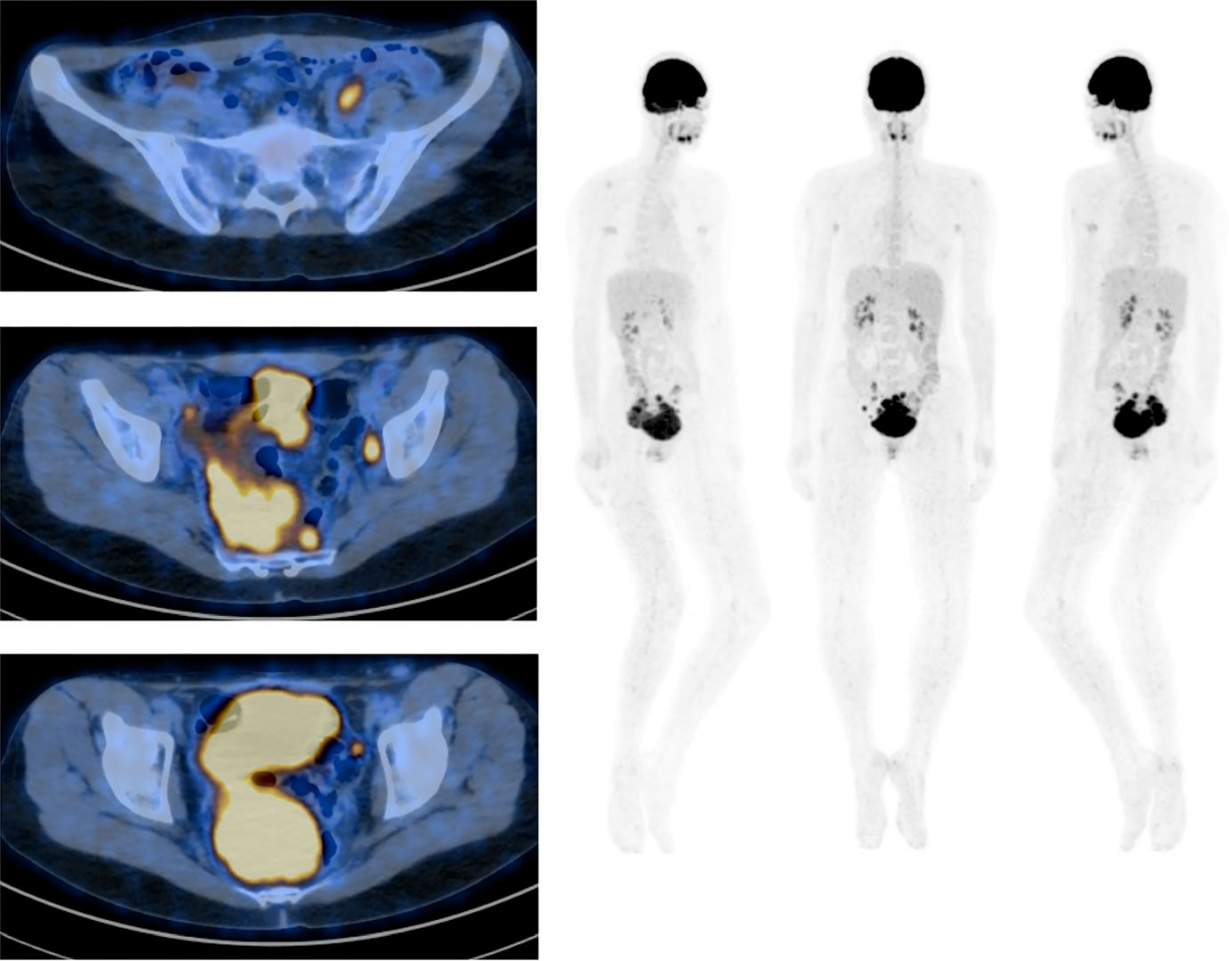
Positron emission tomography/computed tomography scan shows a big mass in the pelvis and multiple smaller nodes in the peritoneum (27 July 2019)
